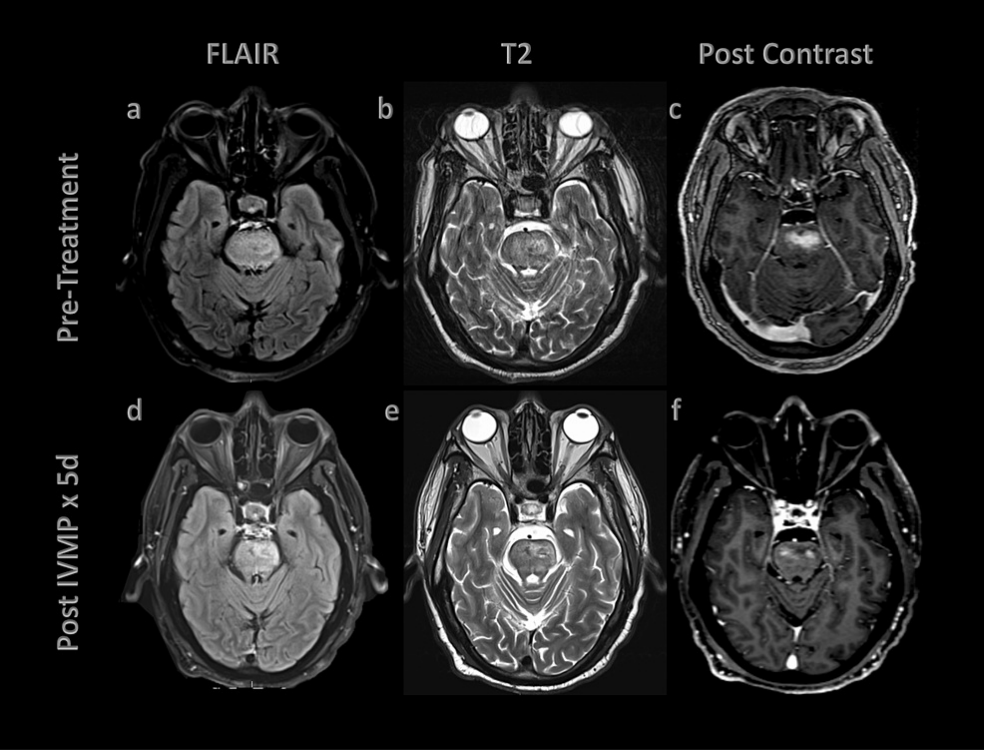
Before and after response to steroids in CLIPPERS patient as demonstrated on axial postcontrast T1-weighted MRIs (a) Axial fluid-attenuated inversion recovery (FLAIR) image shows expansile hyperintense lesion involving the pons. (b) Axial T2-weighted image shows intermediate signal intensity in the pons. (c) Axial postcontrast T1-weighted image shows enhancing lesion involving the pons. (d) FLAIR imaging shows a slight reduction of hyperintense lesions compared to prior imaging. (e) Axial T2-weighted image shows unchanged intermediate signal intensity in the pons. (f) Five days after treatment with IVMP, axial postcontrast T1-weighted image shows reduced enhancement of the pons. CLIPPERS - Chronic lymphocytic inflammation with pontine perivascular enhancement responsive to steroids
